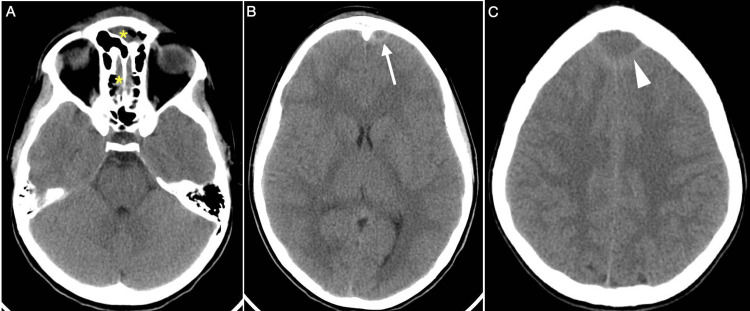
CT scan with no contrast of Pott’s puffy tumor. (A) A CT scan with no contrast displayed sinusitis of the ethmoidal and frontal sinuses (yellow asterisk). An epidural collection of pus is noted near the inner frontal protuberance (white arrow) and superior sagittal sinus (arrowhead) in images (B) and (C), respectively.
